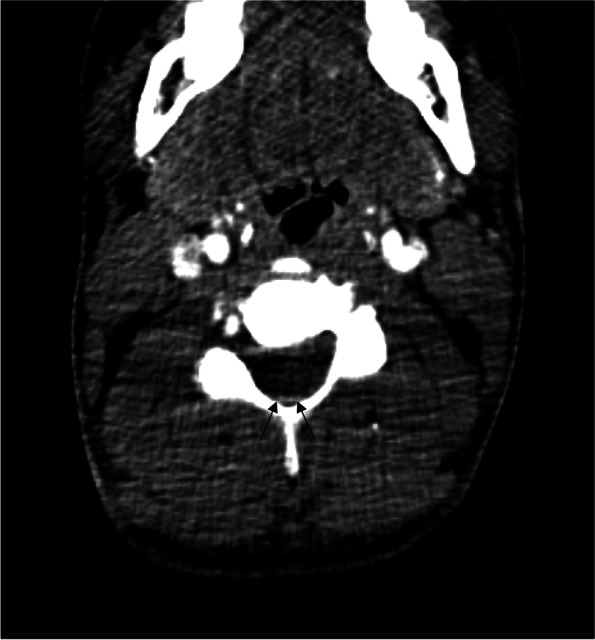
CT aortogram, arterial phase, C5 level. Arrows pointing to subtle hyperdensity
